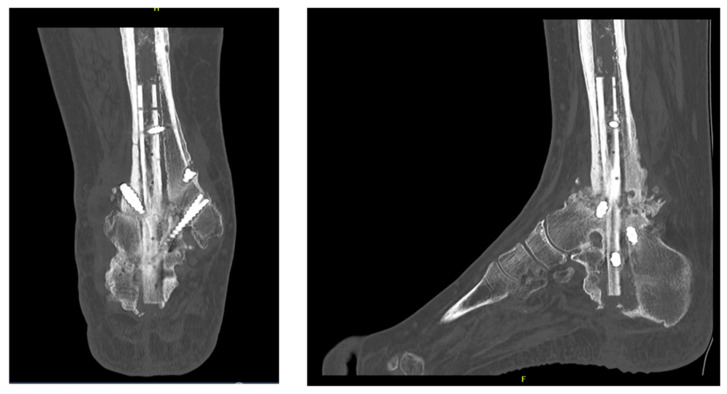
Computed tomography (CT) scan obtained 3 months after revision TTC re-arthrodesis, shown in coronal and sagittal views.
